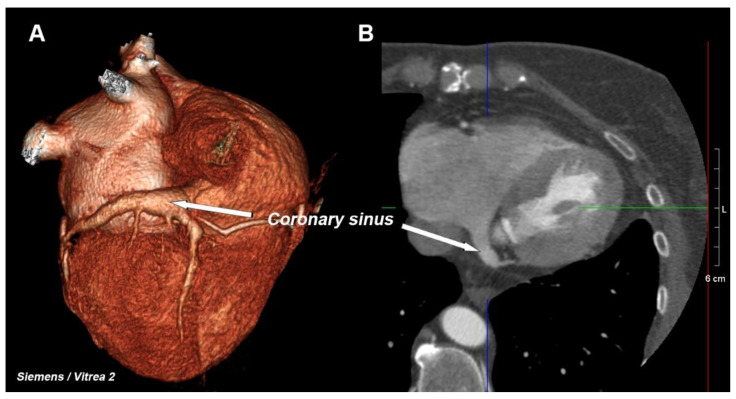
Example of CT image of the heart with coronary sinus visible. (A) 3D volume rendering. (B) 2D MPR multiplanar reformatted reconstruction.
